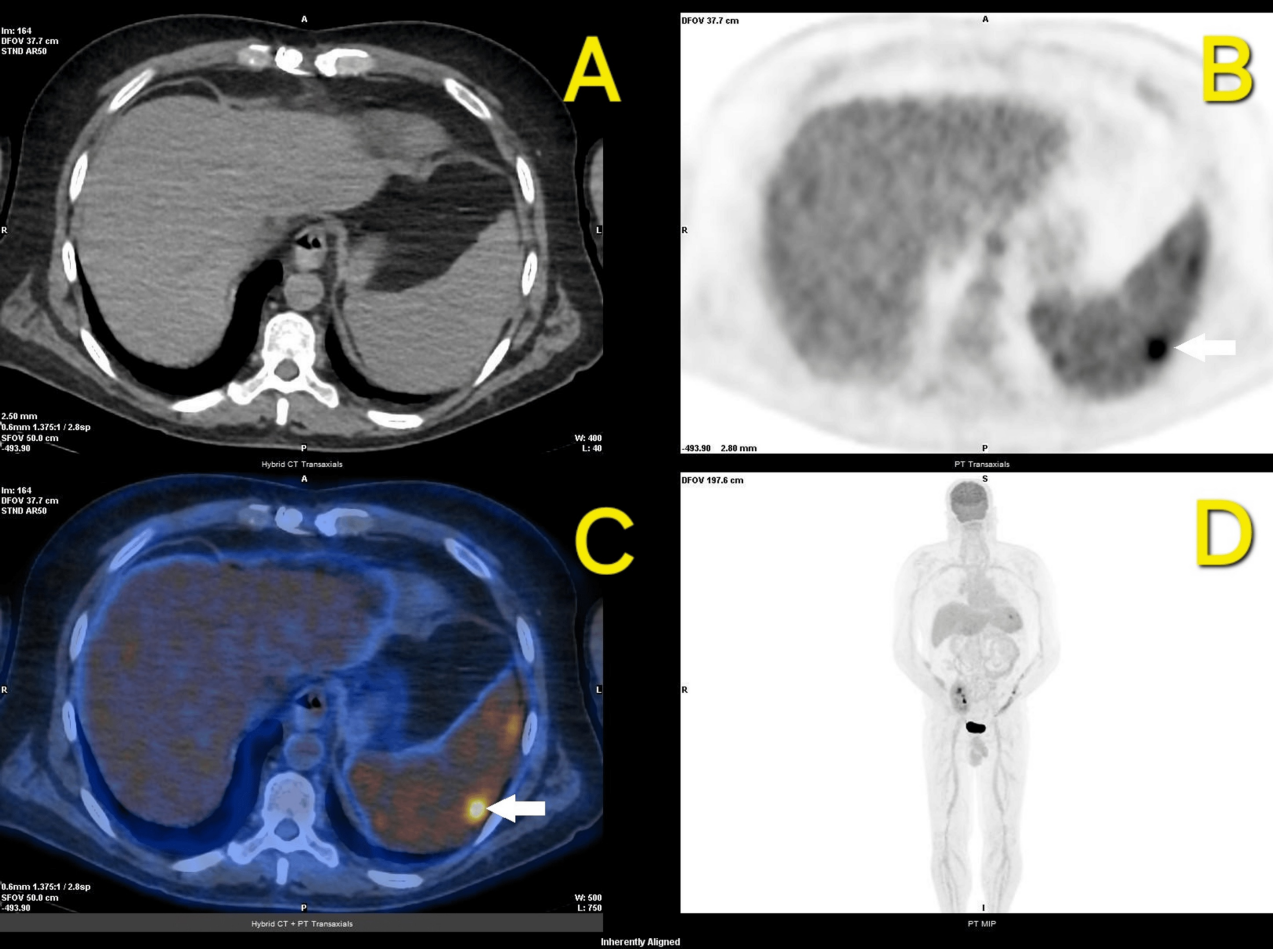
PET-CT with 18F-FDG showing diffusely increased splenic uptake with a nodular, avid lesion in its periphery (white arrows). (A) axial CT; (B) axial PET; (C) axial PET-CT fusion; (D) PET maximum intensity projection (MIP) PET: positron emission tomography;  18F-FDG: fluorodeoxyglucose F 18
